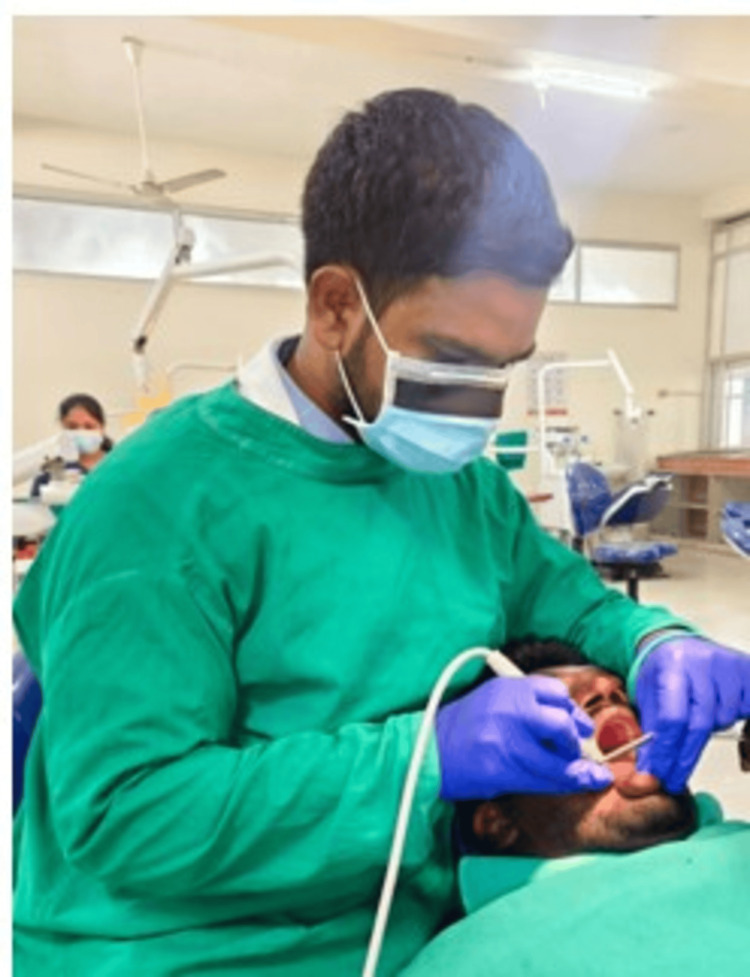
Ultrasonic scaling performed without magnification.
